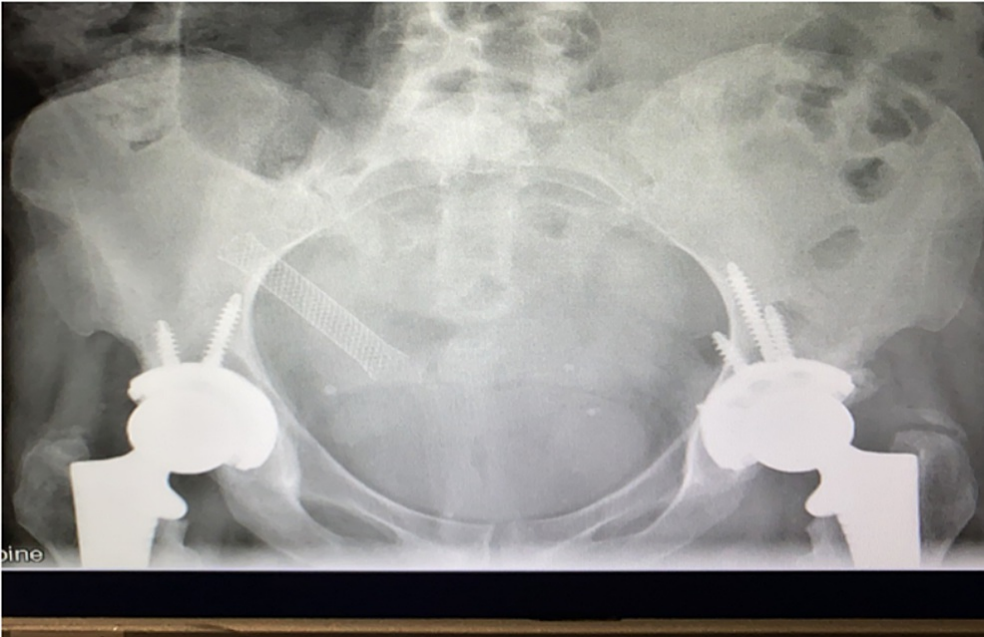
Pelvis X-ray with bilateral prostheses in situ
